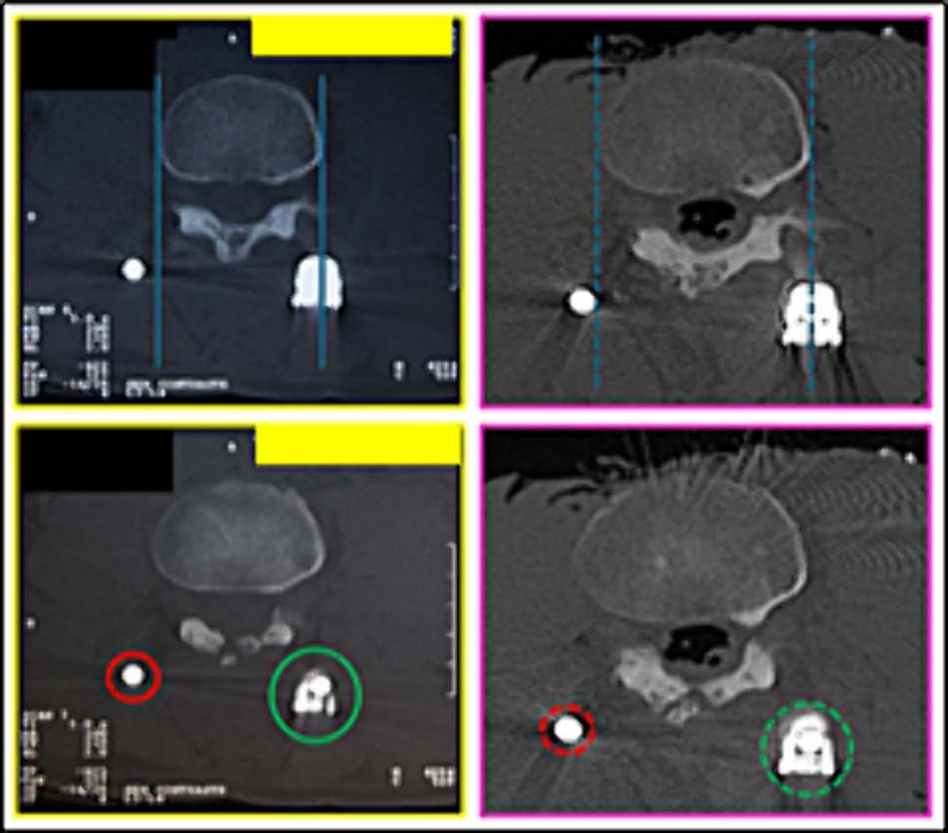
L3 vertebral body. Comparison between the axial slice images of the antemortem (left) and postmortem (right) CT scans. Consistent elements.
